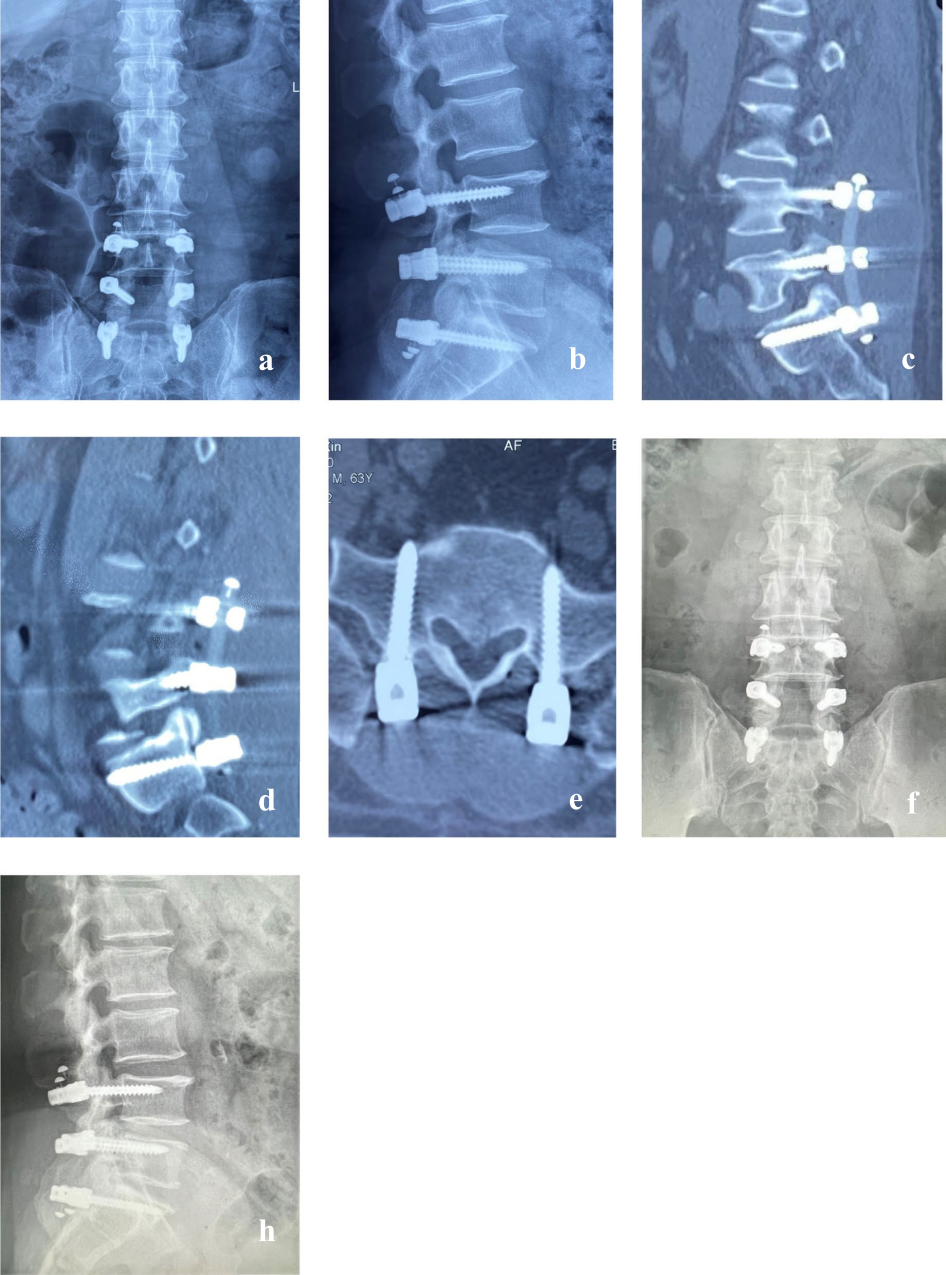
Postoperative Day 7, anteroposterior and lateral X-ray images (a, b) showed satisfactory screw fixation and appropriate depth of sacral screw placement. Postoperative Day 7, lumbar spine CT scans (c, d, e) revealed bilateral sacral screws achieving bicortical fixation. Postoperative Month 28, anteroposterior and lateral X-ray images (f, h) demonstrated well-maintained positions of bilateral sacral screws, with no evidence of loosening or fracture
